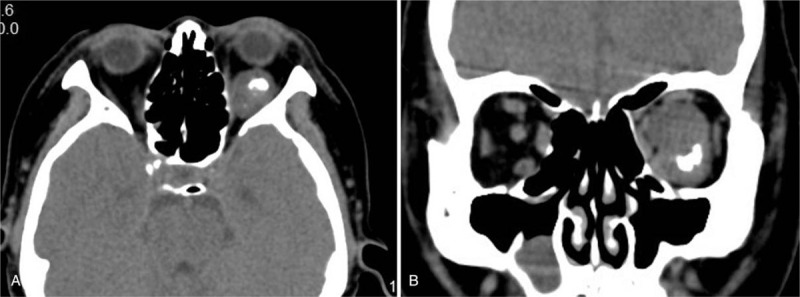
The images showed a well-defined mass in the left orbit muscle cone with a hyperdense speck suggestive of coarse calcification. (A) Axial CT scans. (B) Coronal CT scans.
